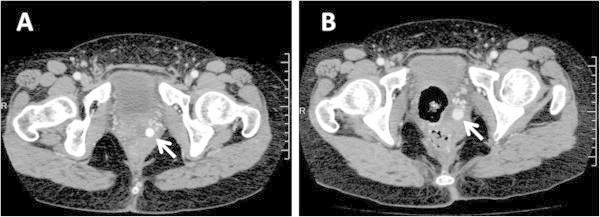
Pelvic contrast-enhanced computed tomography pseudoaneurysms (white arrows). (A) on the fourth postpartum day (B) on the sixth postpartum day.
